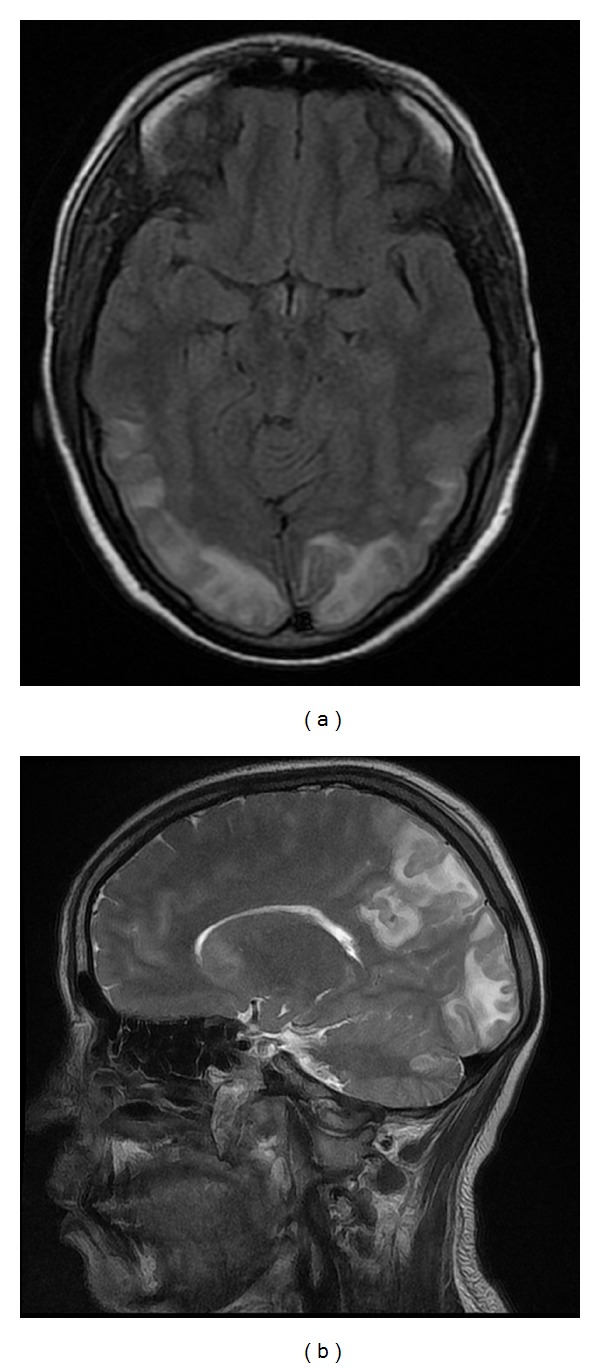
The patient MRI findings in postoperative first day.
